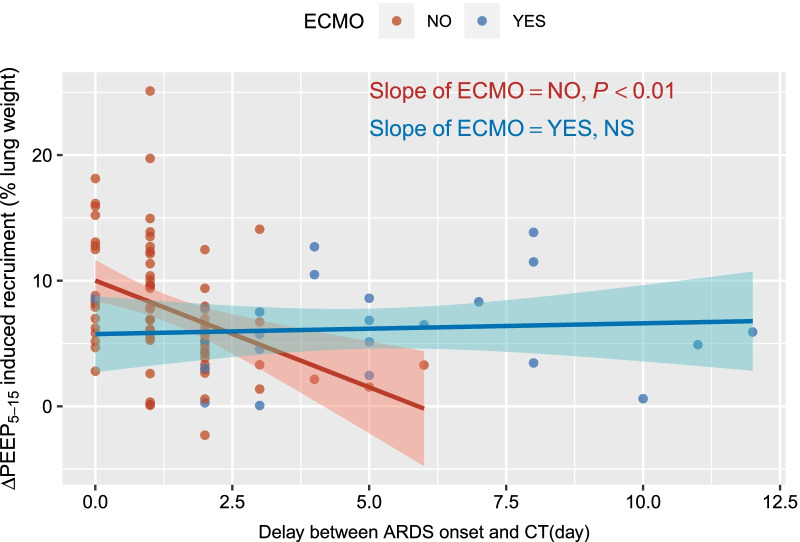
Relationship between ∆PEEP_5-15_-induced recruitment and delay between ARDS onset and CT, according to ECMO status. Each point is partial residuals of the multivariate model with ∆PEEP_5–15_-induced recruitment as the dependent variable, and the following independent variables: EELV at PEEP 5 cmH_2_O, poorly inflated lung at PEEP5 and the interaction between ECMO status × delay between CT and ARDS onset. Lines are regression lines according to ECMO status. Shadowed areas are 95% confidence interval for each regression line. ARDS, acute respiratory distress syndrome, CT, computed tomography, ECMO, extracorporeal membrane oxygenation, ∆PEEP_5-15_-induced recruitment, amount of recruitable lung between PEEP 5 and 15 cmH_2_O, EELV, end-expiratory lung volume, NS, non-statistically significant, PEEP, positive end-expiratory pressure
